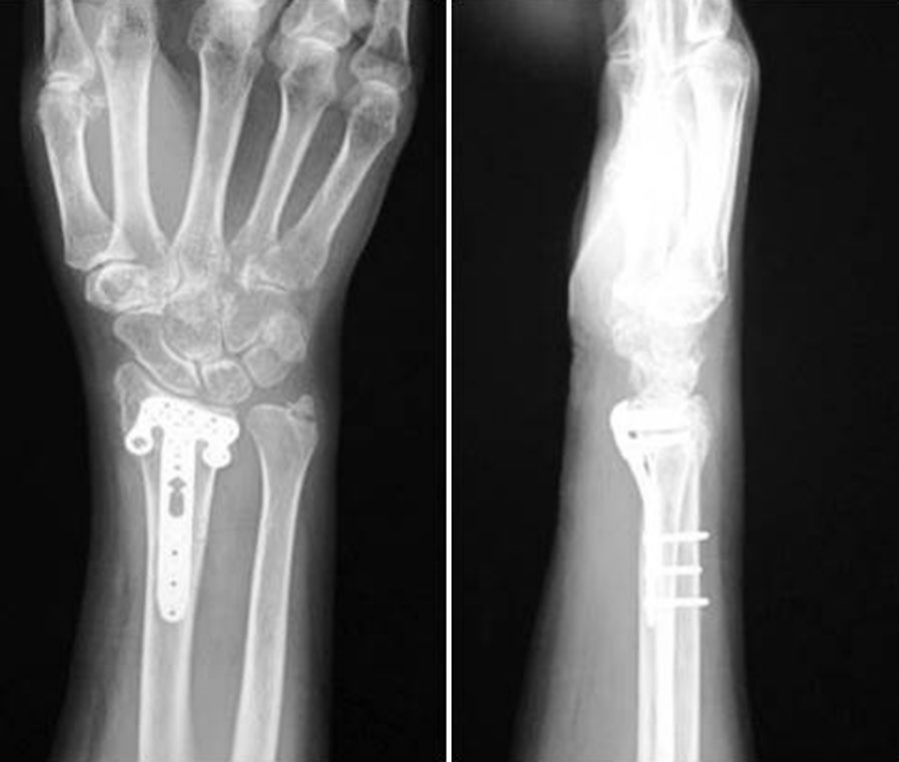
X-rays 4 months after surgery showing good reduction and stabilization with the locking plate for the rim fragment
